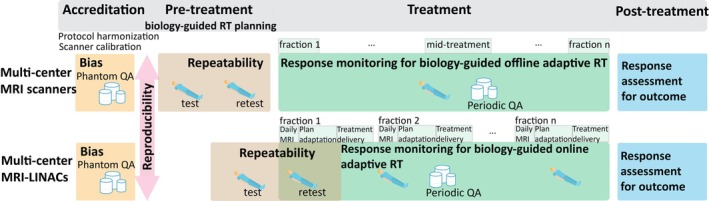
Multicenter clinical trial design framework for the development and validation of quantitative imaging biomarkers for biology‐guided radiation therapy (RT). The framework can be applied to studies using MRI scanners as well as MR‐linear accelerators (MRI‐LINACs).
